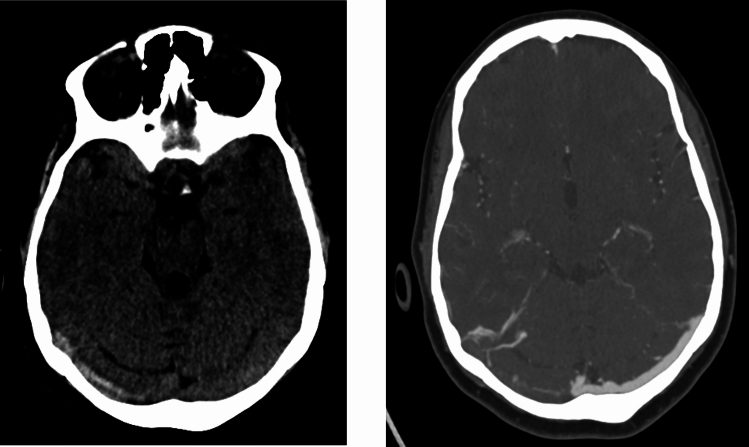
Hyperdense Sinus Sign. Non-contrast CT demonstrates hyperdense sinus sign in the right transverse sinus (left panel) and corresponding thrombosis on CT-venography (right panel)
